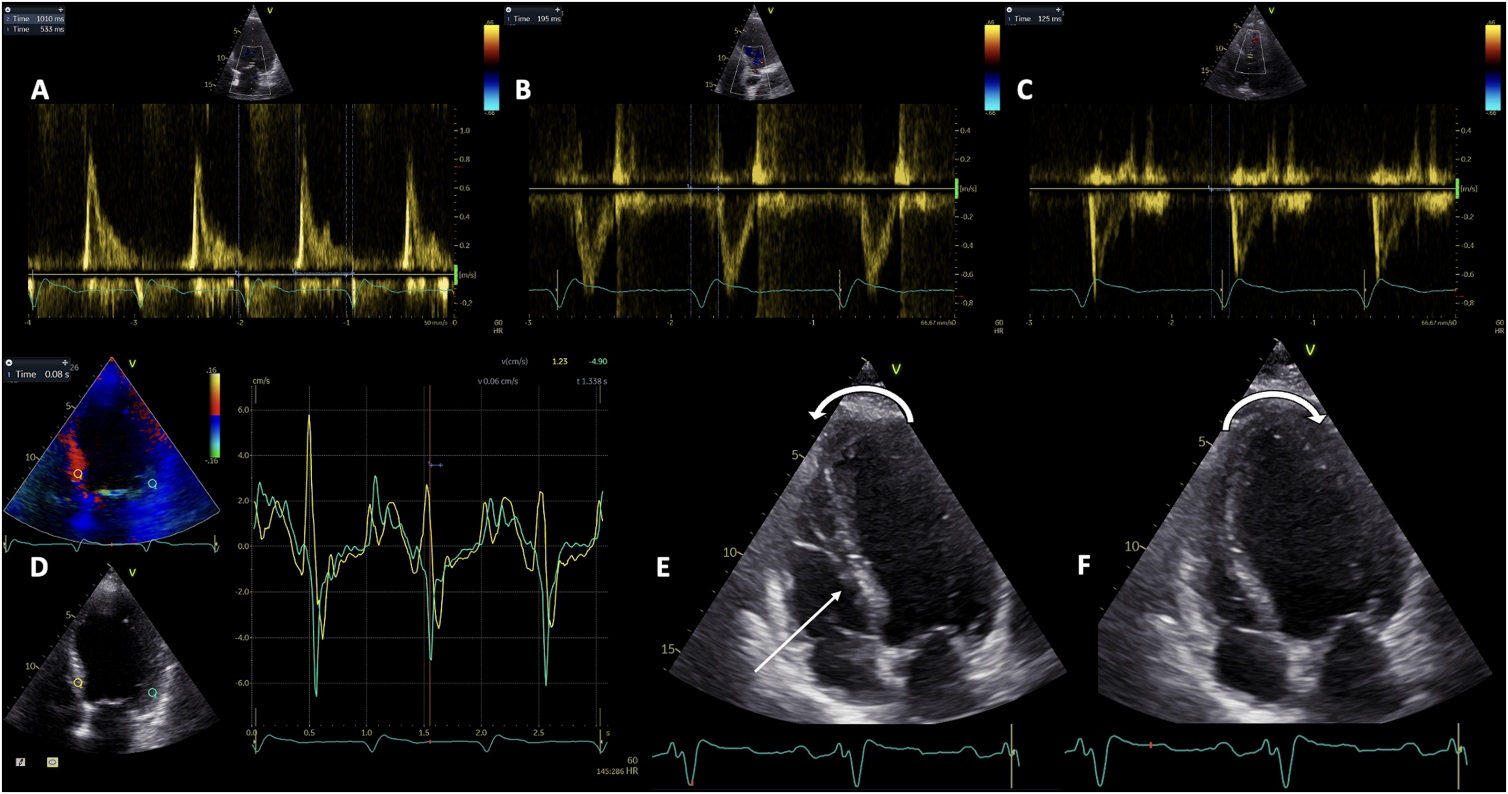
The evaluation of the three types of mechanical dyssynchrony by Doppler echocardiography. Pulsed-wave Doppler assessment of atrioventricular dyssynchrony as the diastolic filling time relative to cardiac cycle duration (A); and interventricular dyssynchrony as the difference between LV pre-ejection time (B), and RV pre-ejection time (C) Tissue Doppler Imaging evaluation of intraventricular dyssynchrony as the basal septal to lateral wall delay (D) Representation of septal flash and apical rocking (E and F). LV, left ventricle; RV, right ventricle.
